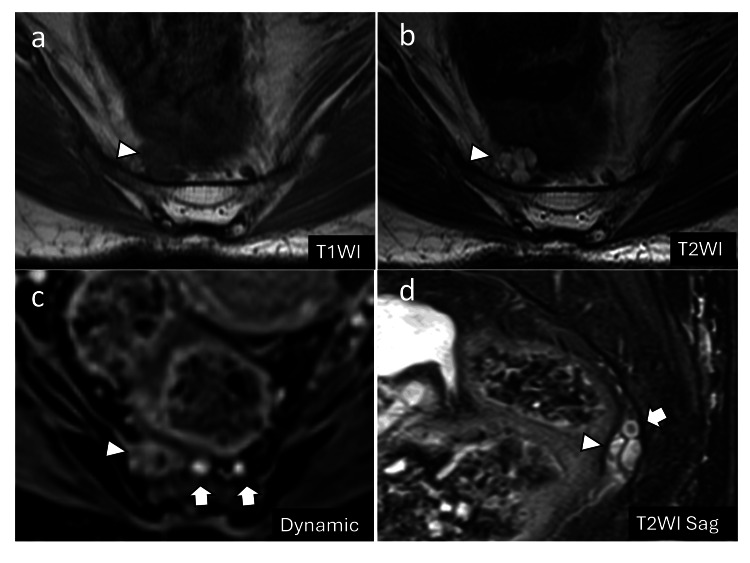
Preoperative MRI (a, b) MRI showing a multilocular cystic mass with low signal intensity on T1-weighted images and high signal intensity on T2-weighted images (arrowheads). (c, d) Contrast-enhanced MRI revealing the median sacral artery and vein (arrows) located adjacent to the mass (arrowheads). MRI: magnetic resonance imaging
